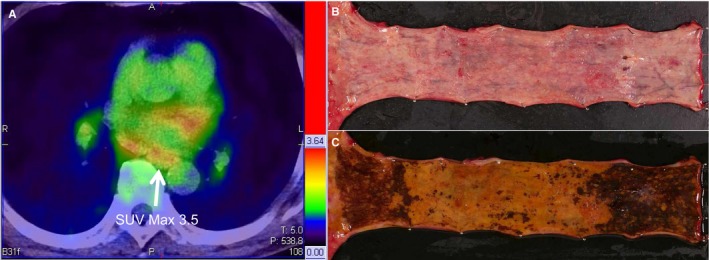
A case of superficial extensive spreading‐type SCC. A, FDG‐PET image. FDG uptake (SUV
